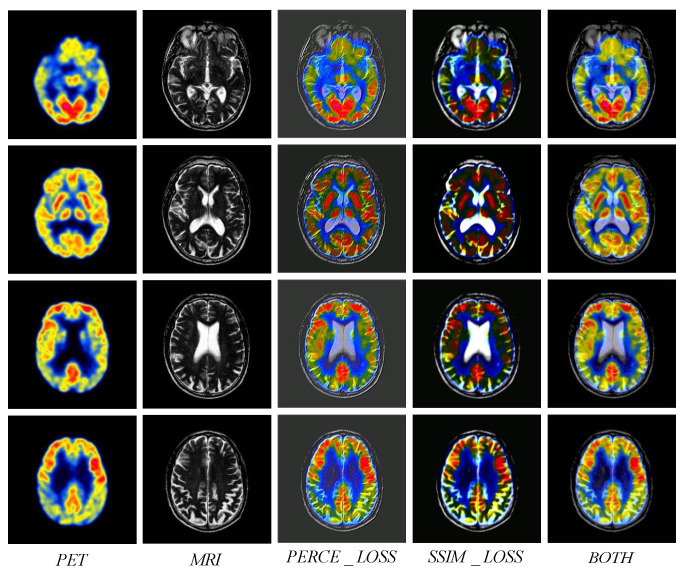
Fusion results under different loss constraints. From left to right: PET and MRI images, only perceptual loss, only SSIM loss, and using both perceptual loss and SSIM loss.
